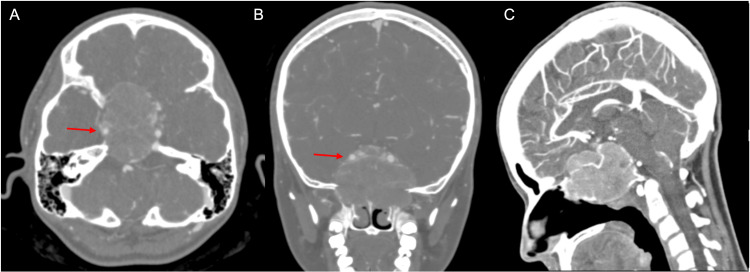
CT angiography images in (A) axial, (B) coronal, and (C) sagittal planes show mild narrowing of the right cavernous ICA (red arrows), arterial enhancement of the mass and multiple prominent vessels traversing the mass. ICA: Internal carotid artery.
